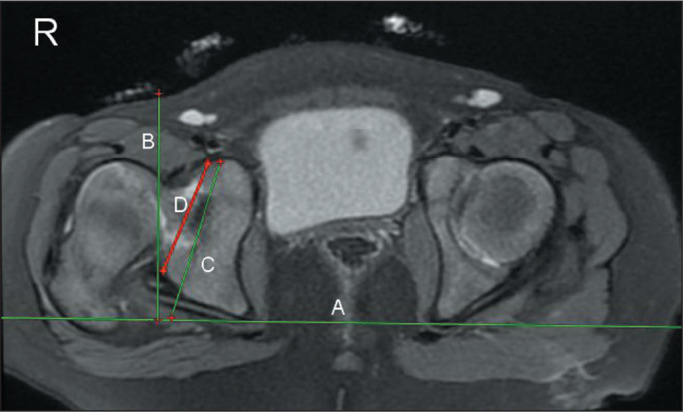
Axial intermediate-weighted fat-suppressed proton density FSE MRI
